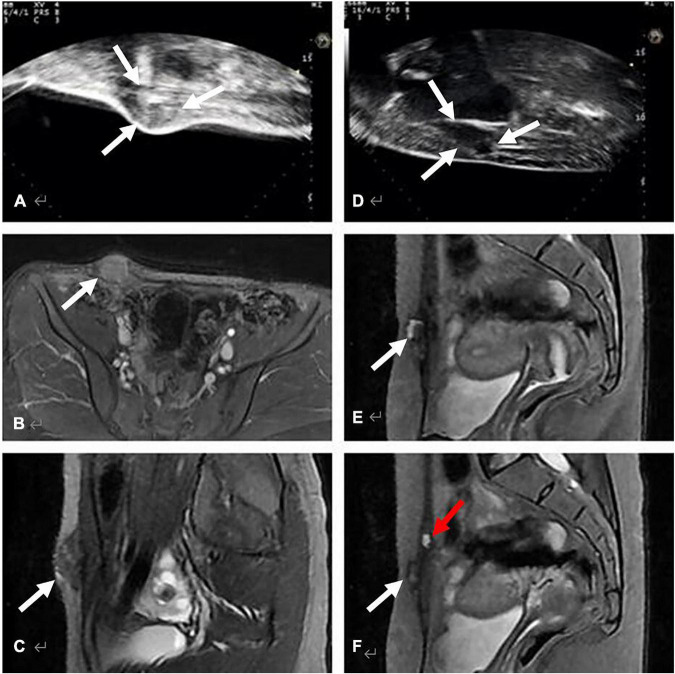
Comparison of AWE lesions between MRI and color Doppler ultrasound before HIFU treatment. (A–C) show an AWE lesion on the right horn of the scar, located in subcutaneous fat, invaded the skin and the anterior sheath of the right rectus abdominis: (A) Long-axis sonogram showed a hypoechoic, nodular mass (white arrow). (B,C) show the axial and sagittal T2-weighted MRI of the same lesion, MRI images were clearer than ultrasound images in terms of size and boundary of the AWE lesion. (D–F) show AWE in a 27-year-old woman with complaints of cyclical pain symptoms after cesarean section, due to the presence of 5 cm of abdominal wall fat. Ultrasound images (D) revealed only one lesion, while MRI clearly revealed two lesions, along with the location and shape of AWE (E,F).
